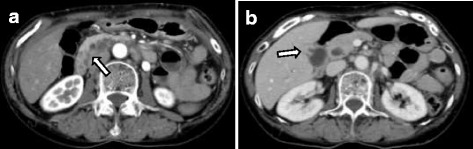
Abdominal computed tomography (CT). a Low-density mass and cystic lesions in the pancreatic head. b Thickness in the wall adjoining the bottom of the gallbladder
